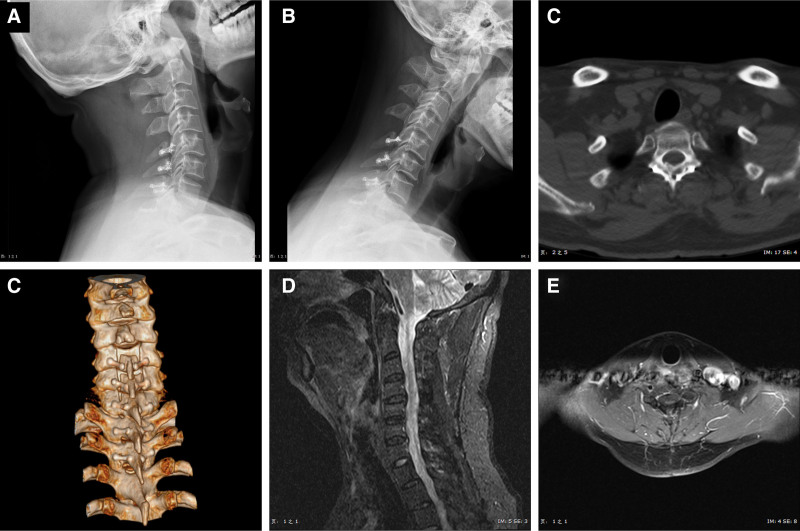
Follow-up 4 yr after surgery. (A, B) The dynamic X-ray examination verified that the spinal stability was preserved. (C, D) The CT scan showed that the bone fusion happened within the lamina-spinous process-ligament complex. (D, E) The sagittal and axial enhanced MRI images showed the recurrency by tumor did not happen. CT = computed tomography, MRI = magnetic resonance imaging.
